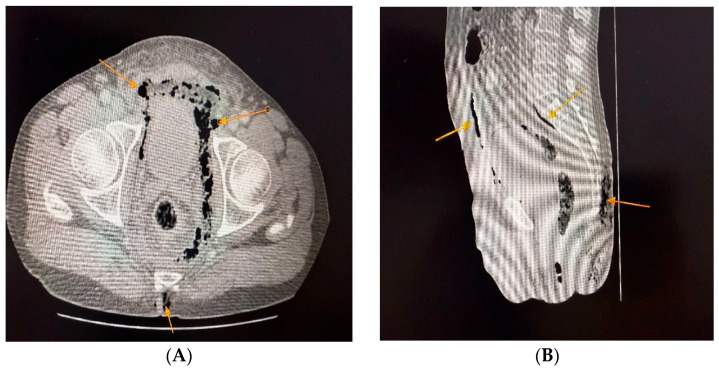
The CT scan demonstrates evidence of pro- and retropneumoperitoneum (A-yellow arrow), as well as a loculated collection with gas within the ischiorectal fossa, suggestive of an advanced infectious process with tissue necrosis (B-yellow arrow).
